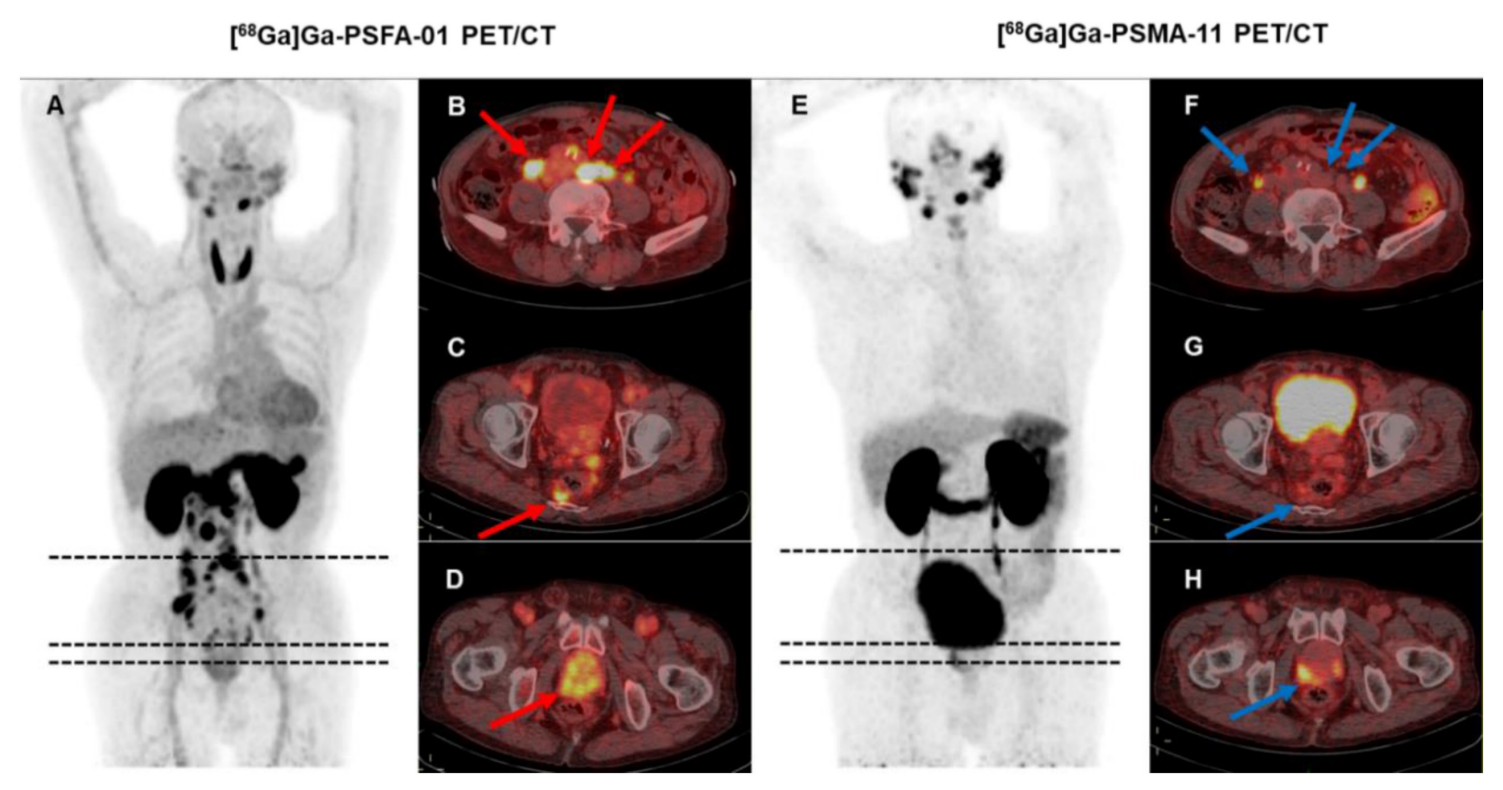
A 74-year-old man (Patient #3) with a Gleason score of 4+4 and a current serum tPSA level of 86.00 ng/mL. [^68^Ga]Ga-PSFA-01 PET/CT showed a greater number of lesions (A-D, red arrows) and more intense tracer uptake than [^68^Ga]Ga-PSMA-11 PET/CT (E-H, blue arrows). In [^68^Ga]Ga-PSFA-01 PET/CT scans, para-abdominal lymph nodes and mesenteric metastases exhibited positive results (B, C), whereas in [^68^Ga]Ga-PSMA-11 PET/CT scans, these findings were absent (F, G).
